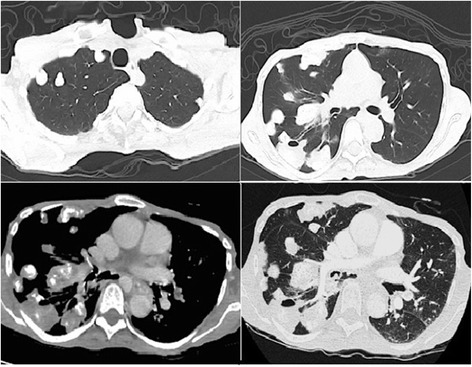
Thorax computed tomography scan showing multiple calcified pulmonary nodules up to 50 mm in diameter, some of which were cavitated
